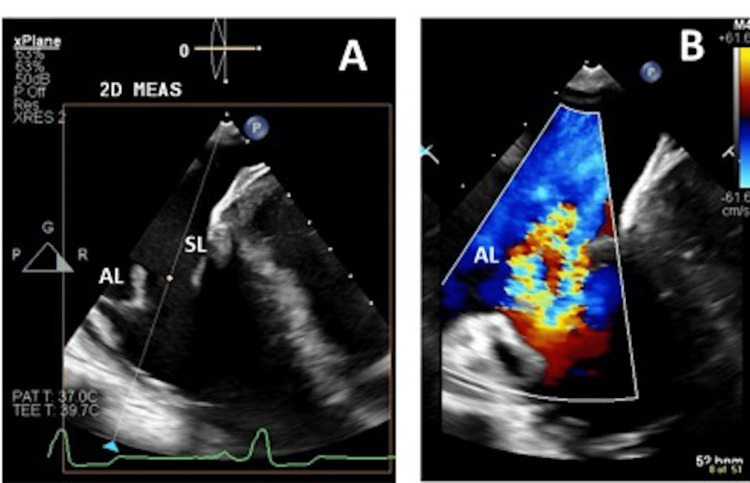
Still-frame images of transesophageal echocardiogram of the tricuspid valve regurgitation. A. Four-chamber view showing the flail of anterior leaflets of tricuspid valve; B. Color comparison images demonstrating the severe tricuspid regurgitation through the coaptation gap AL: anterior leaflet; SL: septal leaflet
